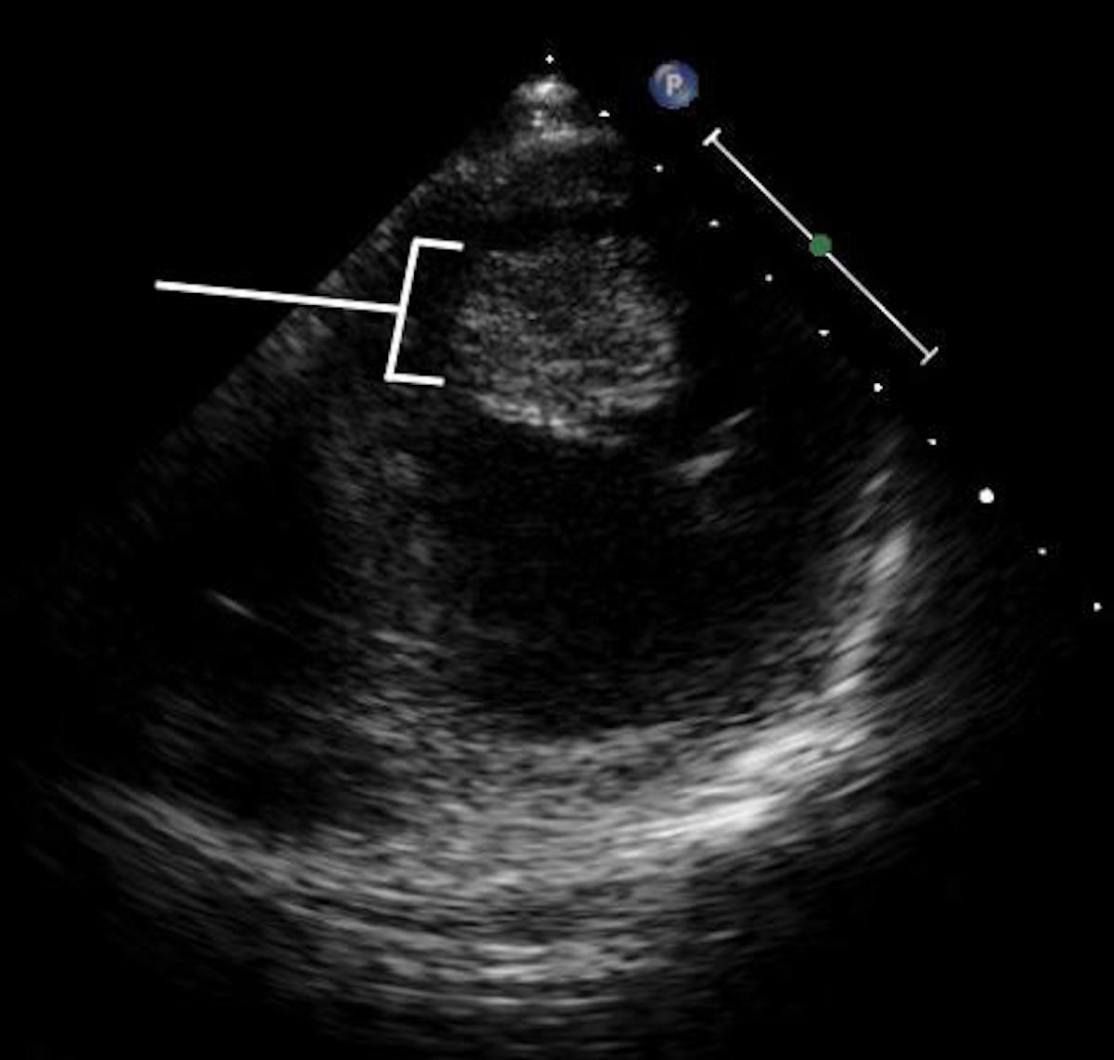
Transesophageal echocardiogram showing the myxoma attaching to the interatrial septum.
